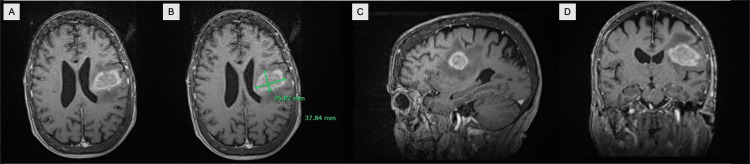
Preoperative MRI. A: T1-weighted MRI with contrast in the axial plane showing an enhancing mass in the inferior left frontal lobe with vasogenic edema. B: T1-weighted MRI with contrast in the axial plane showing an enhancing mass measuring 25.02mm x 37.84mm in the inferior left frontal lobe with vasogenic edema. C: T1-weighted MRI with contrast in the sagittal plane showing an enhancing mass in the inferior left frontal lobe with vasogenic edema. D: T1-weighted MRI with contrast in the coronal plane showing an enhancing mass in the inferior left frontal lobe with vasogenic edema.
